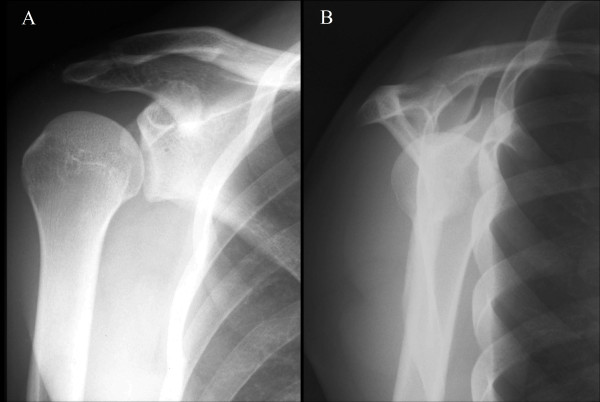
Plain radiographs of the injured shoulder showing normal appearance A, Anteroposterior and B, scapular views of the right shoulder.
